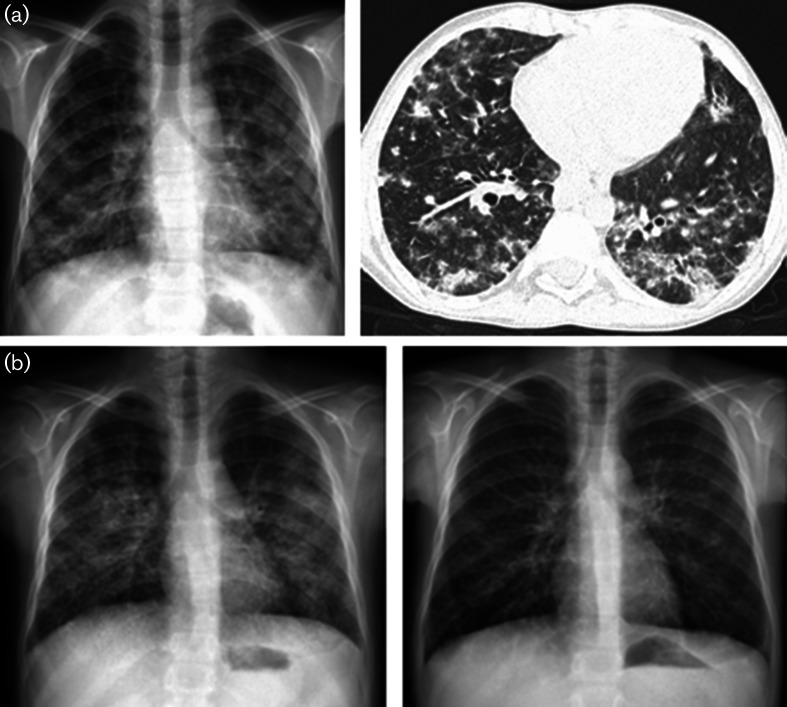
(a) Chest X-ray and thorax CT of Patient 2 (at 10 years of age): bilateral patchy and nodular infiltrations with indistinct margins and coarse septal thickenings. (b) Chest X-ray before and after abatacept therapy (6 months): bilateral hazy parenchymal infiltrations at the start of abatacept therapy. There was almost complete resolution of parenchymal infiltrations with only residual coarse reticular interstitial markings.
